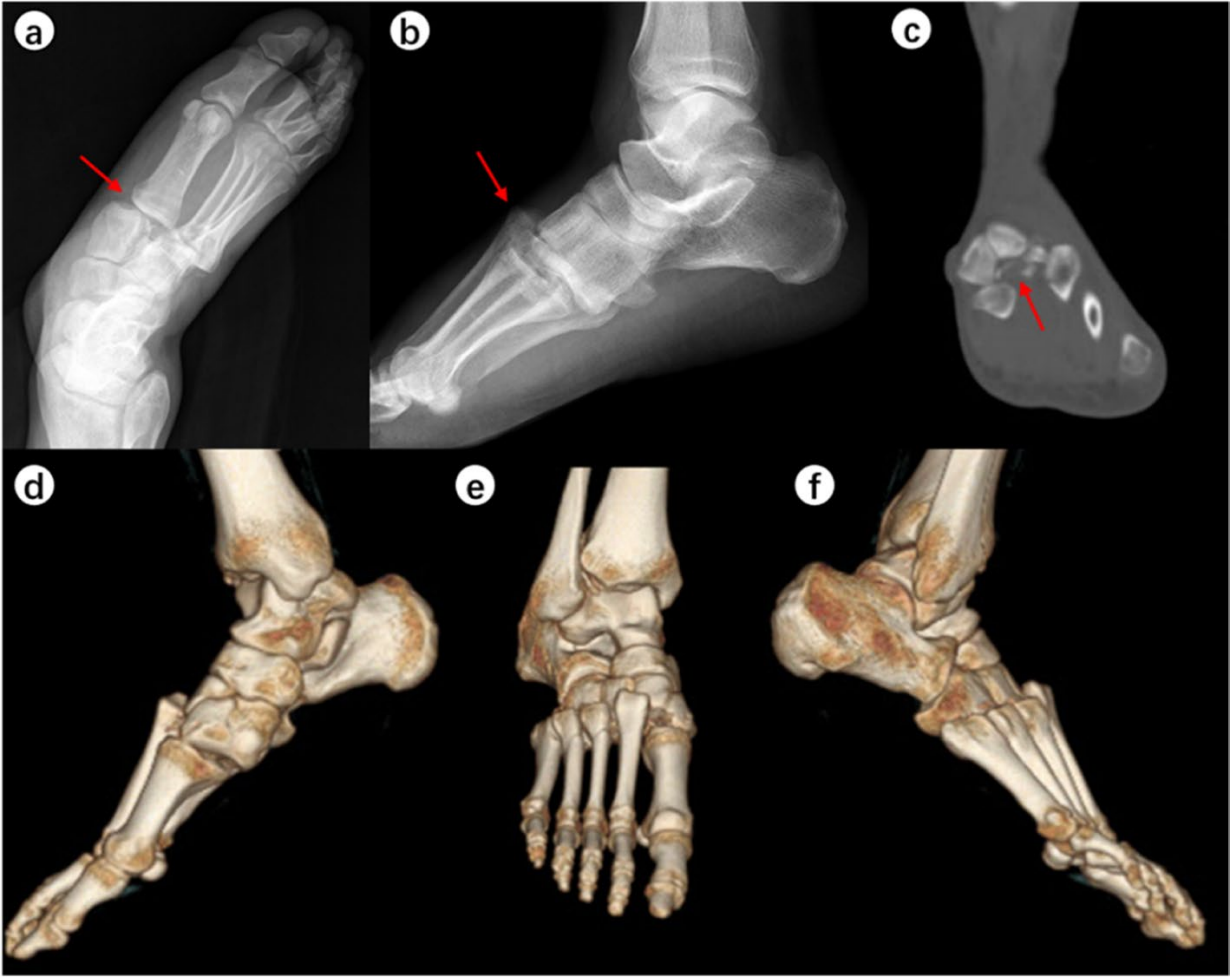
A 42-year-old man had a traffic collision and hurt his right foot. Chiodo-Myerson’s classification: three-column injury; Displacement classification: displaced injury. Both two observers made the correct diagnosis for two times, and didn’t change the initial treatment option (surgery) after evaluating CT image. a-b The conventional radiographs showed obvious tarsometatarsal joint dislocation (red arrows). It was easily diagnosed. c-f CT unraveled more details: intra-articular fractures of the base of the second and third metatarsal bone as well as extensive dorsal-lateral dislocation of the tarsometatarsal joint. The red arrow indicates the fracture fragments
